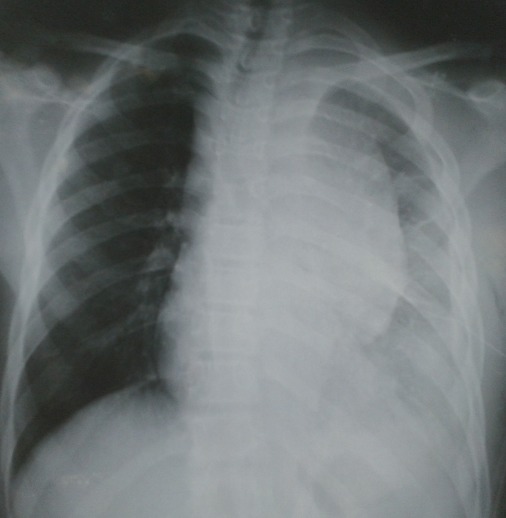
Radiographie thoracique montrant une énorme masse médiastino pulmonaire gauche
